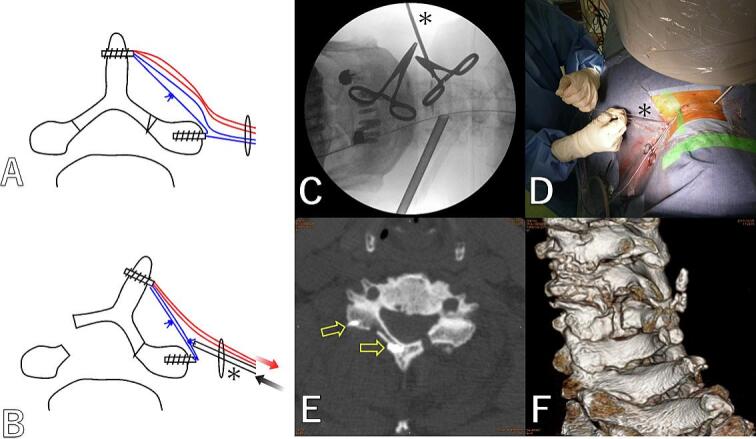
Minimally invasive ODL under full-endoscope. A: Suture anchors fixed to the base of the spinous process and the lateral side of the lateral mass. B: While maintaining the opening of lamina by pulling the thread, another thread was tied outside the skin incision, and the knot was tightened using a knot pusher (asterisk) to complete the fixation. C: Fluoroscopic image. D: While maintaining the opening of lamina by pulling the thread, the knot was tightened using a knot pusher to complete the fixation. E: Postoperative CT axial image at C5. The expansion of the lamina reverted. (Anchor: yellow arrow) F: CT 3D image. Laminoplasty was performed on C5 by decompressing C4–6. (For interpretation of the references to colour in this figure legend, the reader is referred to the web version of this article.)
